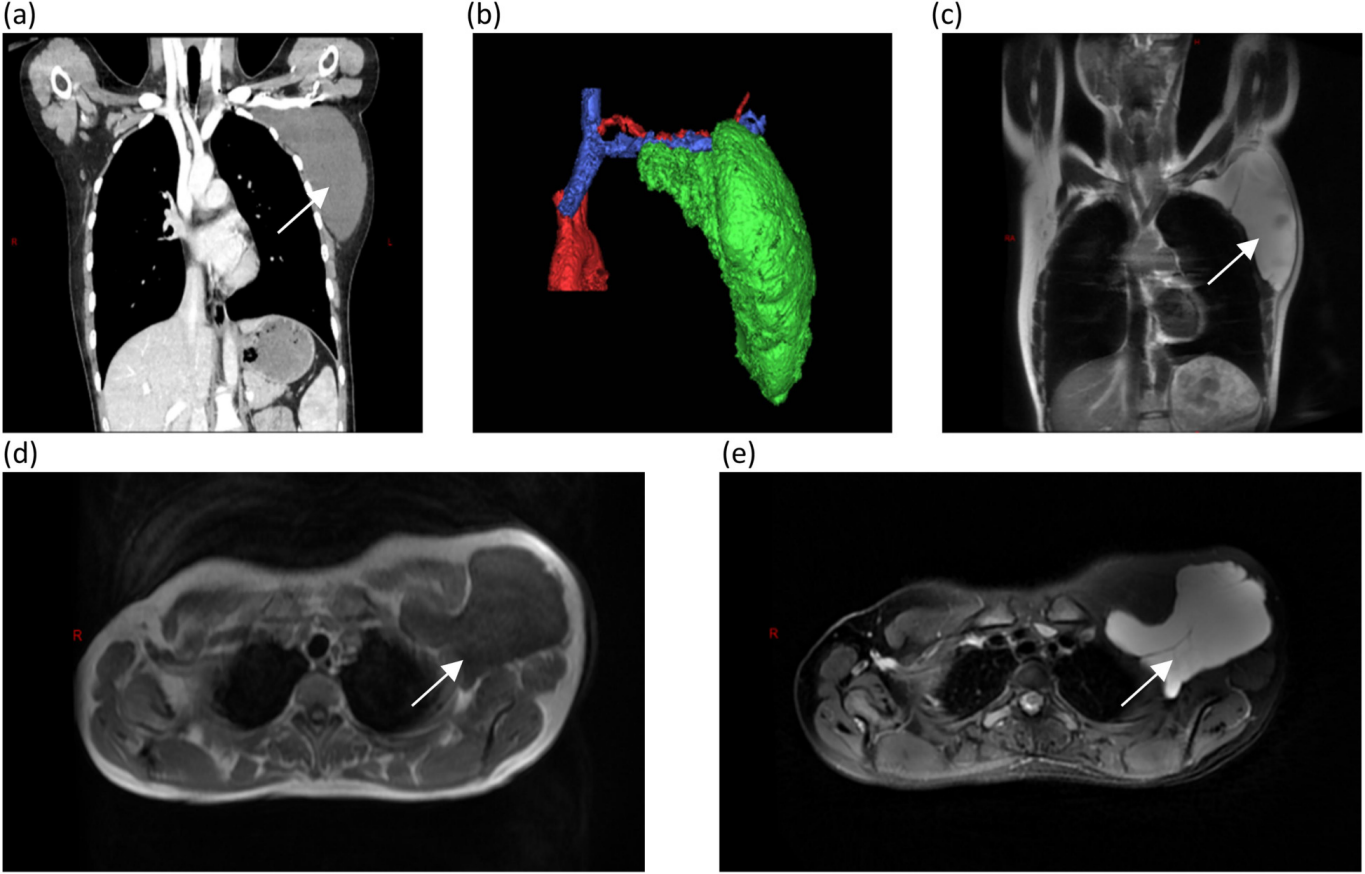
Computed tomography and magnetic resonance imaging, with arrows identifying the mass: (a) CTA revealing a large cystic mass in the left axilla; (b) A three-dimensional reconstruction showing that the mass is adjacent to the subclavian vessels; (c) A coronal view of an MRI examination; and (d,e) long T1 and T2 signals appear hypointense (dark) on T1-weighted images appearing hyperintense (bright) on T2-weighted images.
